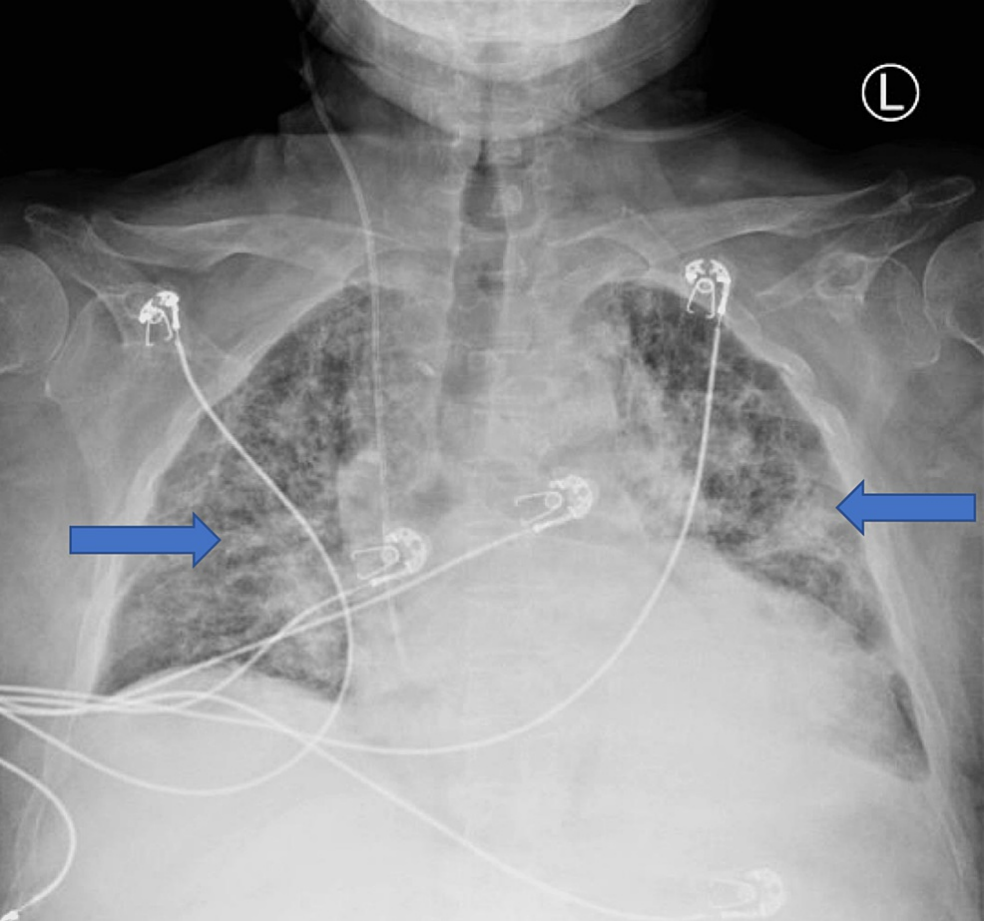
Chest X-ray before cefiderocol treatment. Blue arrows show bilateral interstitial infiltrates.
